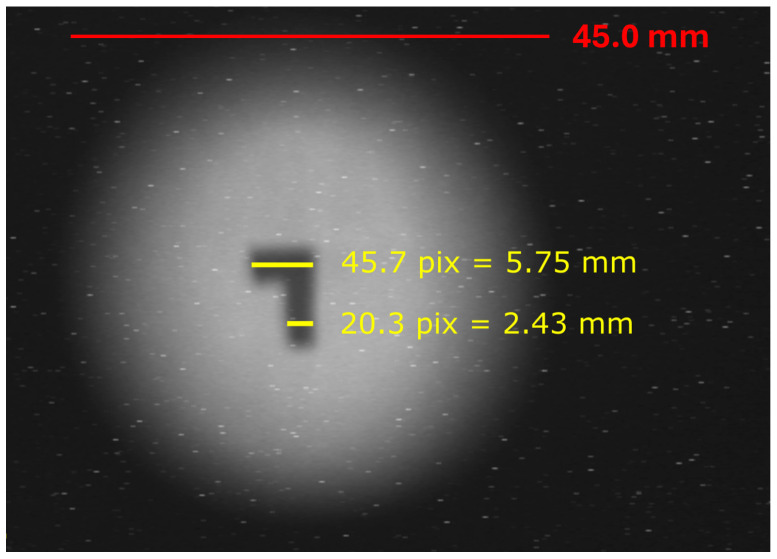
VESUVIO beam profile at thermal energies, obtained via a CCD camera and a neutron-absorbing converter material. The L-shaped darker region at the center of the beam corresponds to the cadmium foil used to calibrate the beam size, with the conversion parameter indicated by the yellow lines. A red bar corresponding to 4.5 cm is given as the size of the beam penumbra. Figure from the instrument internal calibration (2016).
